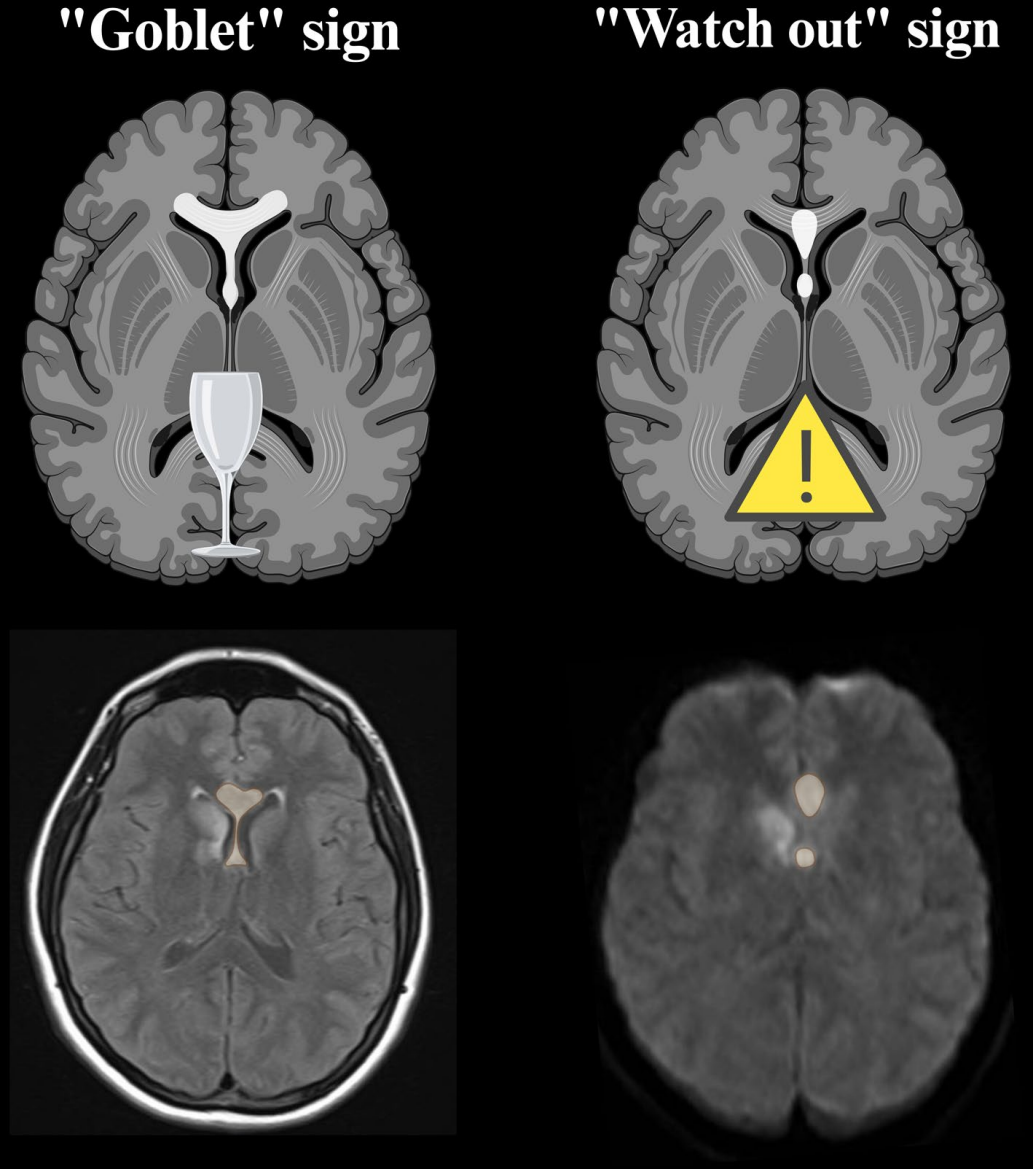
Neuroradiological signs proposed to rapidly recognize the lesional pattern of ScA stroke. They are best visualized on axial DWI sequence in the acute phase (60, 64). The possible concomitant involvement of RAH territory can occur in association with ScA stroke, as can be seen in the MRI images of our cases (created with BioRender.com). DWI, diffusion weighted imaging; ScA, subcallosal artery; RAH, recurrent artery of Heubner.
